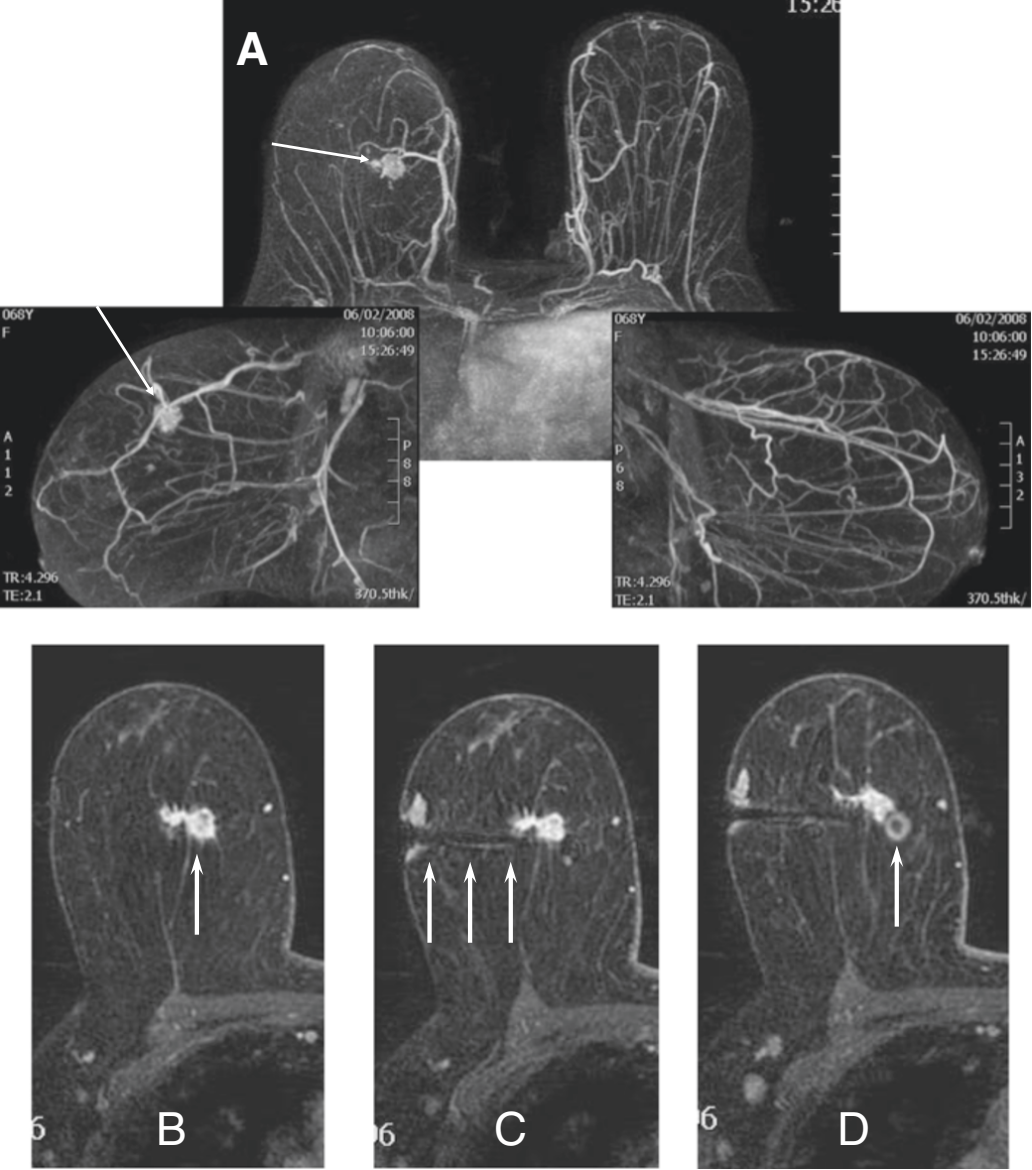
Steps of radiopharmaceutical injection at a suspicious MRI finding. (A) 3D MIP reconstruction contrast-enhanced dynamic study of a bilateral breast magnetic resonance with parallel imaging technology, performed on a suspicious mass in the right breast (arrows). (B) Transverse T1-weighted dynamic contrast-enhanced image showing the upper inner quadrant irregular mass (arrow). (C) Compatible needle near the target lesion (arrows). (D) Perilesional injection reveals an artifact from a small quantity of gadolinium to confirm the location of the injection (arrow). A 52-year-old woman whose excisional biopsy findings revealed a diagnosis of infiltrating ductal carcinoma.
